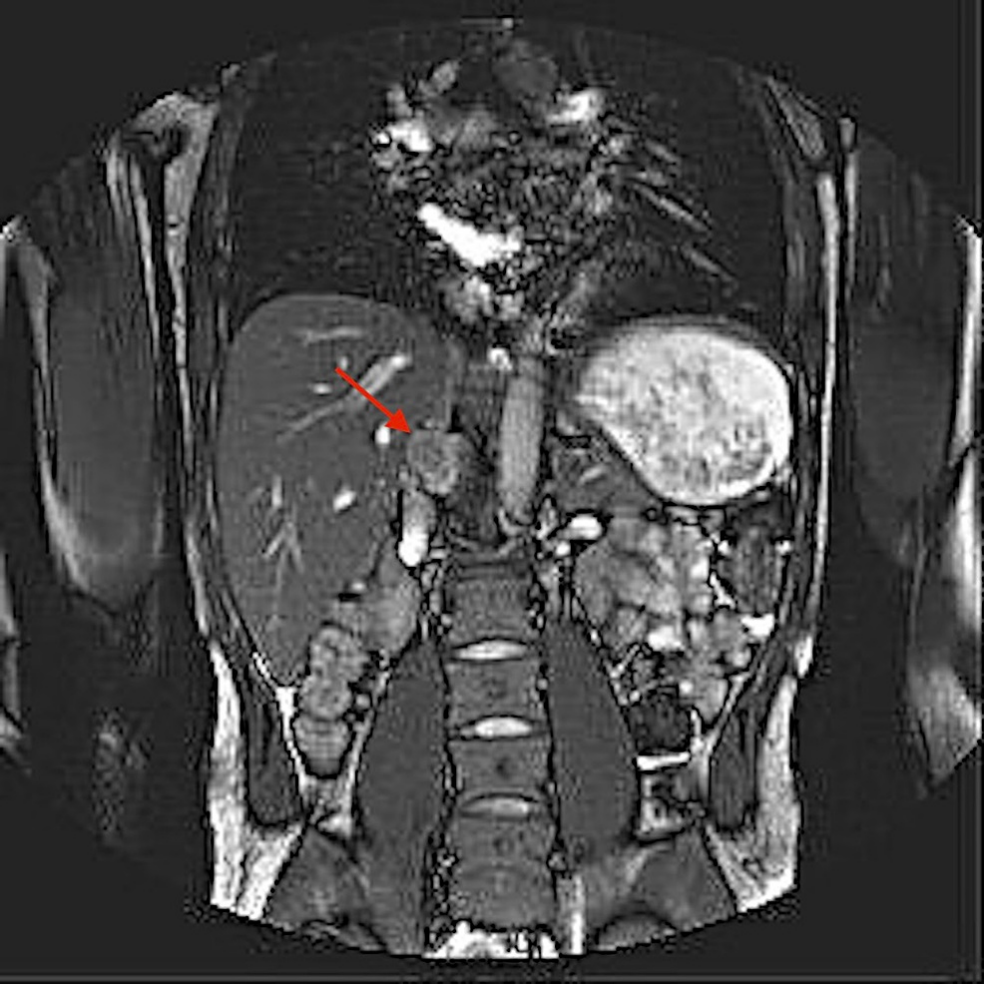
Progressively enhancing mass of 4.7 x 2.7 cm on MRI of the abdomen.
